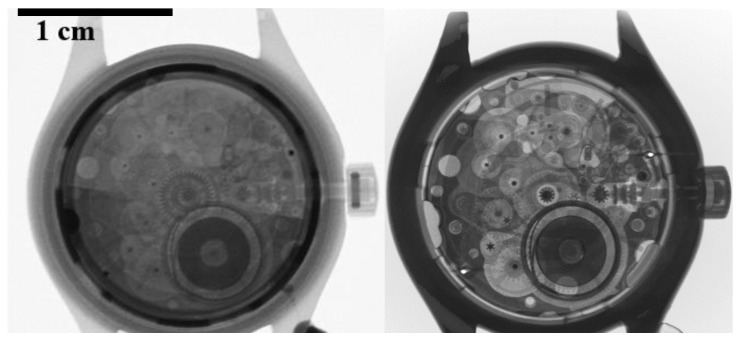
A neutron radiograph (left) and a cut-away of a 3D tomographic reconstruction (right) of a mechanical wristwatch.
